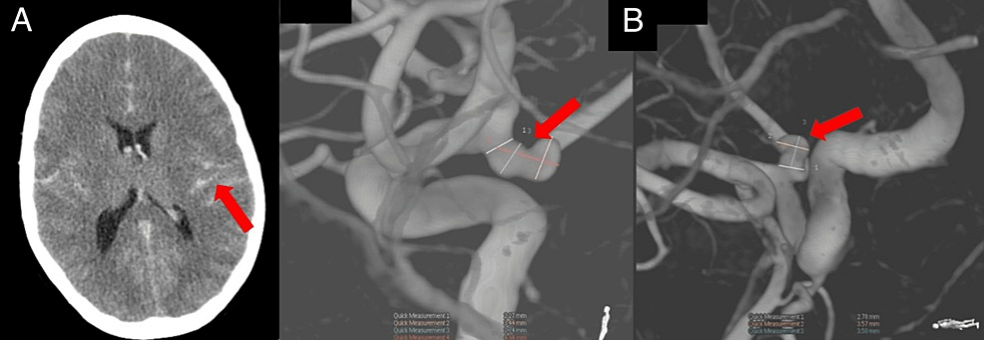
A: Cerebral CT scan on admission showed extensive SAH and diffuse hypodensities compatible with global cerebral edema. B: Cerebral angiography at admission identified an elongated bilobed aneurysm in the left posterior communication artery. SAH, subarachnoid hemorrhage
